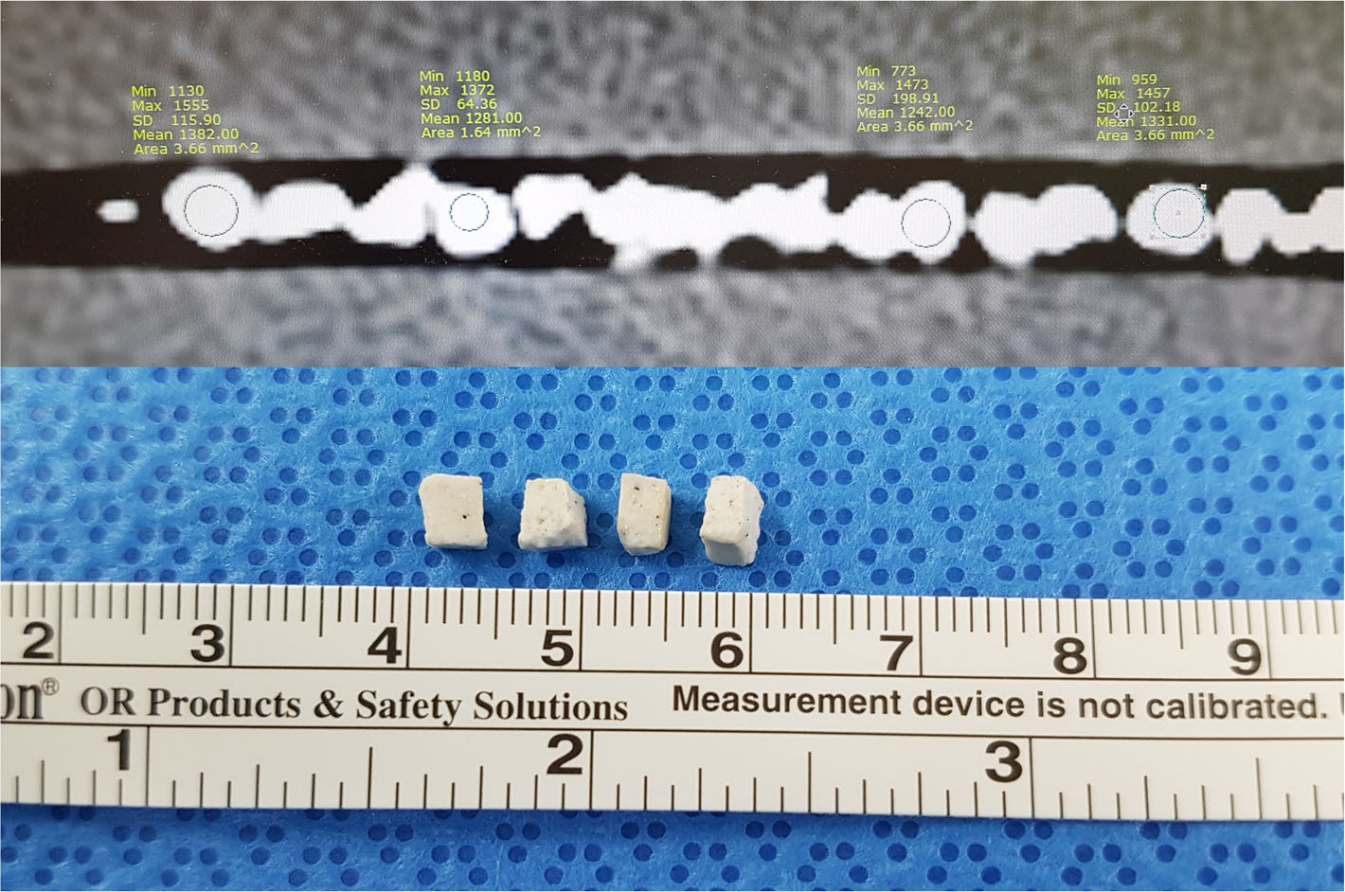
a Stone density measured in the computed tomography scan images. b Each cubical stone of 4x3x3 mm^3^
